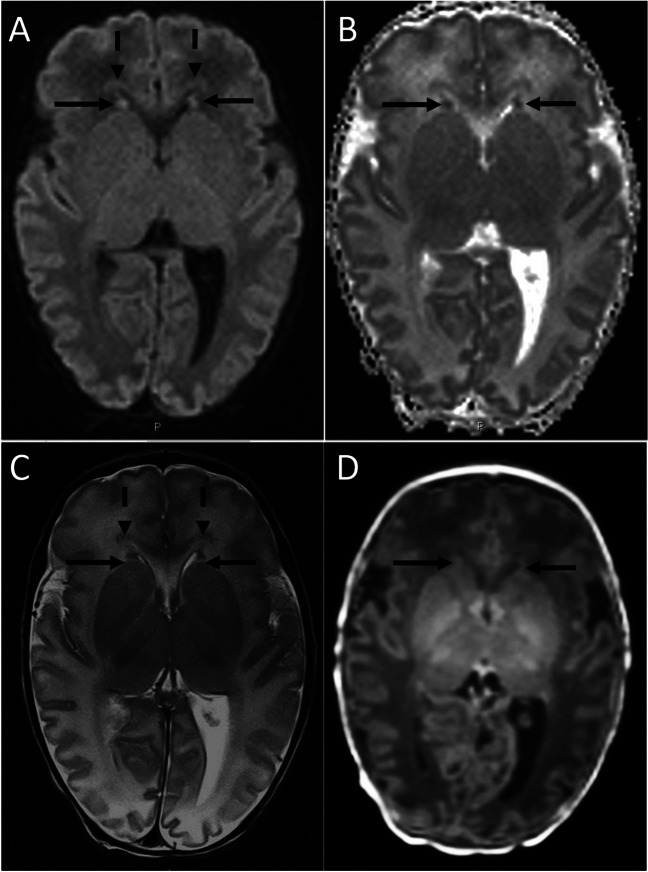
MRI of a newborn scanned at 7 days for suspected seizure activity (35 weeks GA) demonstrating frontal periventricular gray matter (arrows) and migrating bands (dashed arrows) on (A) DWI, corresponding ADC map (B) and T2-Weighted images (C). The DWI signal is higher compared to the nearby white matter, but it is similar to the opercular cortex. Note T1 signal is similar to the cortex (D)
